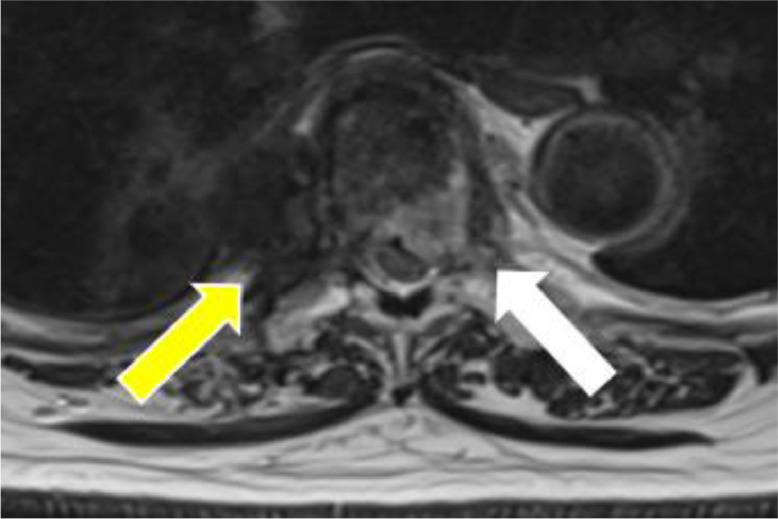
MRI imaging findings. Noncontrast T2-weighted MRI image shows a 35 × 17 × 25-mm mass in Th7-Th9 and a 20 × 24 × 19-mm mass in the Th8 vertebra.
